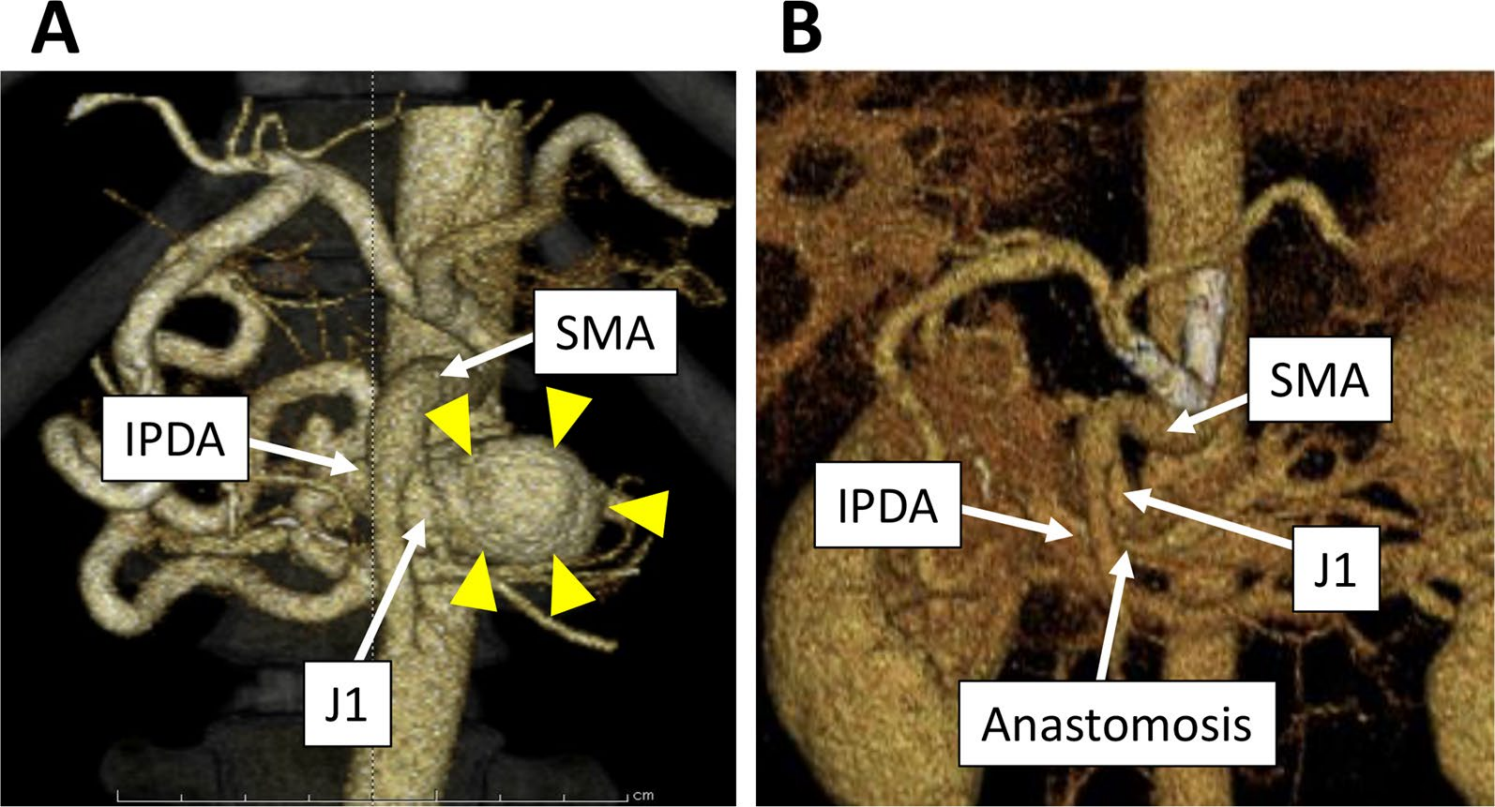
3D-CT finding of the pancreatic arterial arcade in Patient 1.
